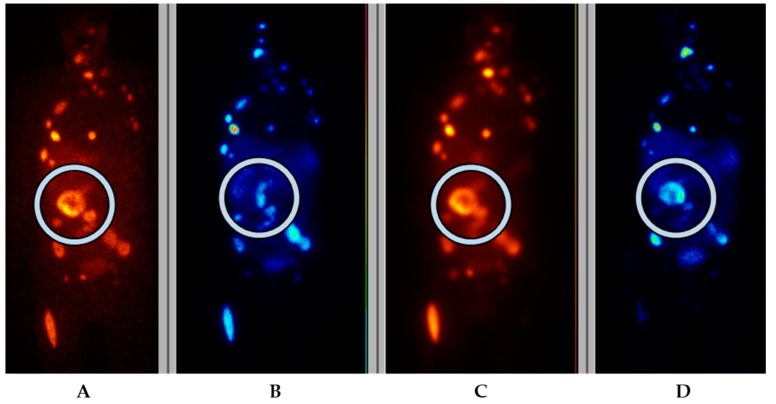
Frontal planar scintigraphy images in patient No. 4, in whom the primary PCC had higher uptake on ^123^I-MIBG scintigraphy (A) than on somatostatin receptor scintigraphy (OctreoScan™) (B). After one cycle of ^131^I-MIBG (C) scintigraphy during therapy) an upregulation of somatostatin receptors was noted and further therapy was given with ^177^Lu-DOTA-octreotate (D) scintigraphy during therapy).
